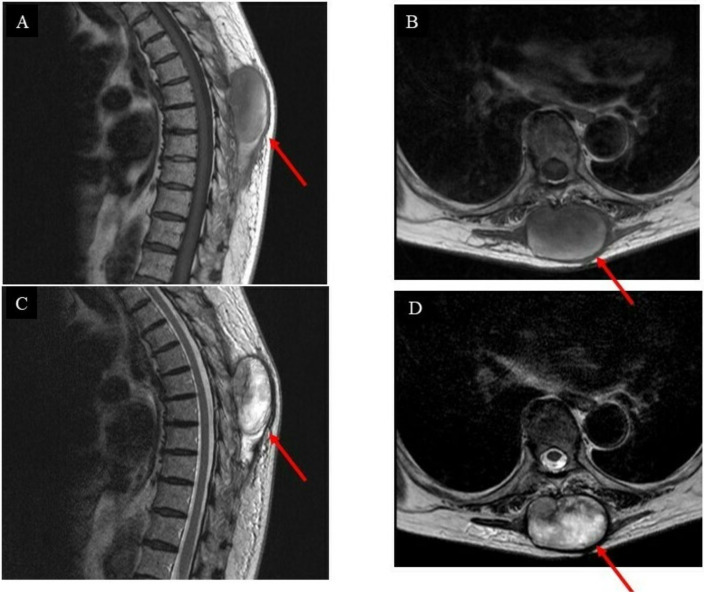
Images of the intrinsic back musculature of the patient three months after wide resection: (A) sagittal magnetic resonance imaging (MRI) T1-weighted sequence; (B) axial MRI T1-weighted sequence; (C) sagittal MRI T2-weighted sequence; (D) axial MRI T2-weighted sequence.
